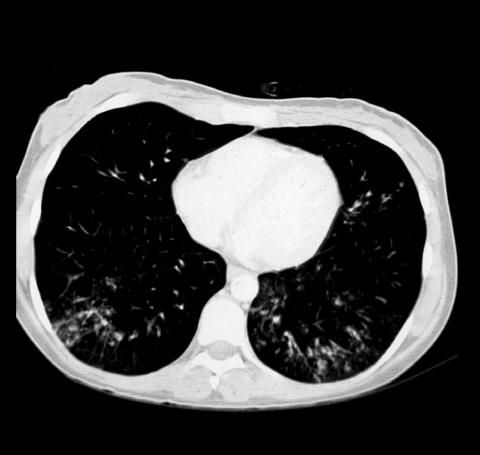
Computed tomography scan showing reticulonodular infiltrations of both lungs in the lower zones.
